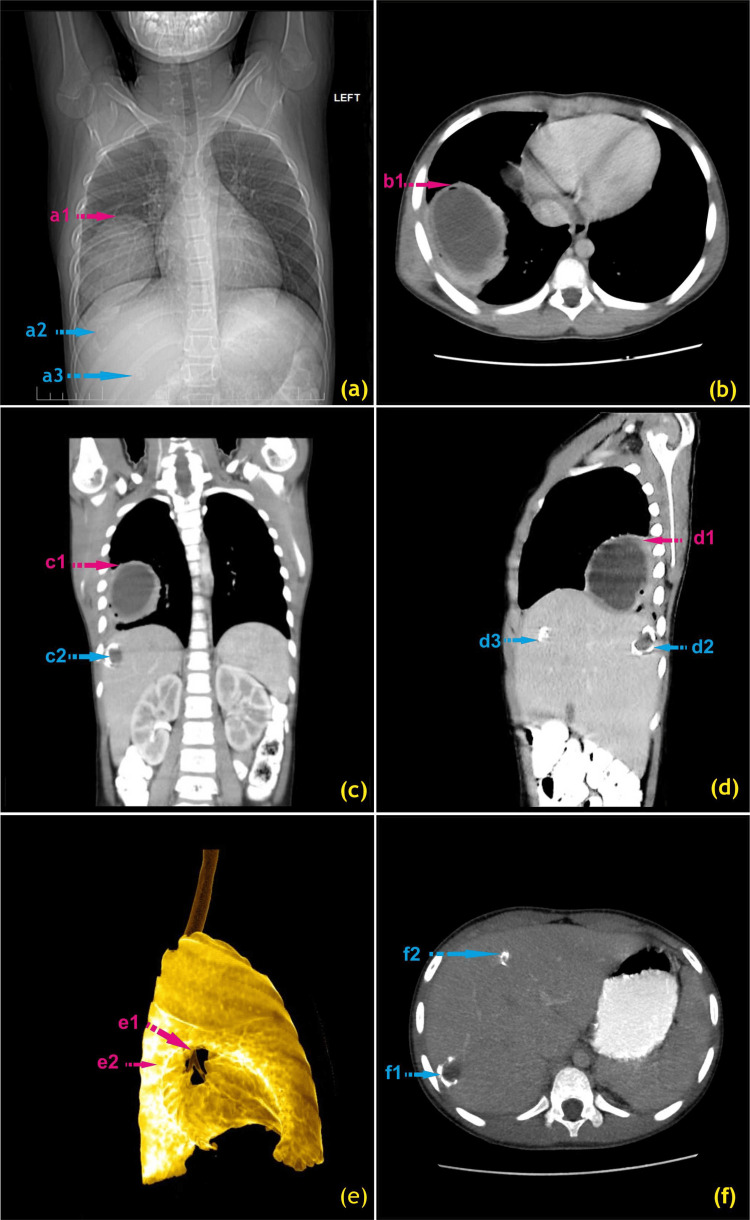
(a) A pre-operative CT scanogram shows big lung opacity, big liver calcification (a2), and small liver calcification (a3). (b) A contrast CT (axial image) shows a double-layered hydatid cyst in the right lower lobe (RLL) with air between layers (b1). (c) A contrast CT (coronal reformation) shows a double-layered lung hydatid cyst in RLL with air between layers (c1) and a calcified liver hydatid cyst in Couinaud segment VII (c2). (d) An oblique sagittal image shows a double-layered lung hydatid cyst with air between layers (d1), a calcified liver hydatid cyst in segment VII (d2), and a small calcified liver cyst in segment IVA (d3). (e) A pseudo-colored minimum intensity projection lateral image of the right lung shows that the lung was destroyed by a lung hydatid cyst (e1) and that the bronchi are intact (e2). (f) Two liver hydatid cysts (axial image) are seen where there is a big calcified hydatid cyst in segment VII (f1) and a small calcified hydatid cyst in segment IVA (f2). Pulmonary hydatid cyst: red marking; hepatic hydatid cyst: blue marking Image credits: Dr. Deepakkumar V. Mehta
